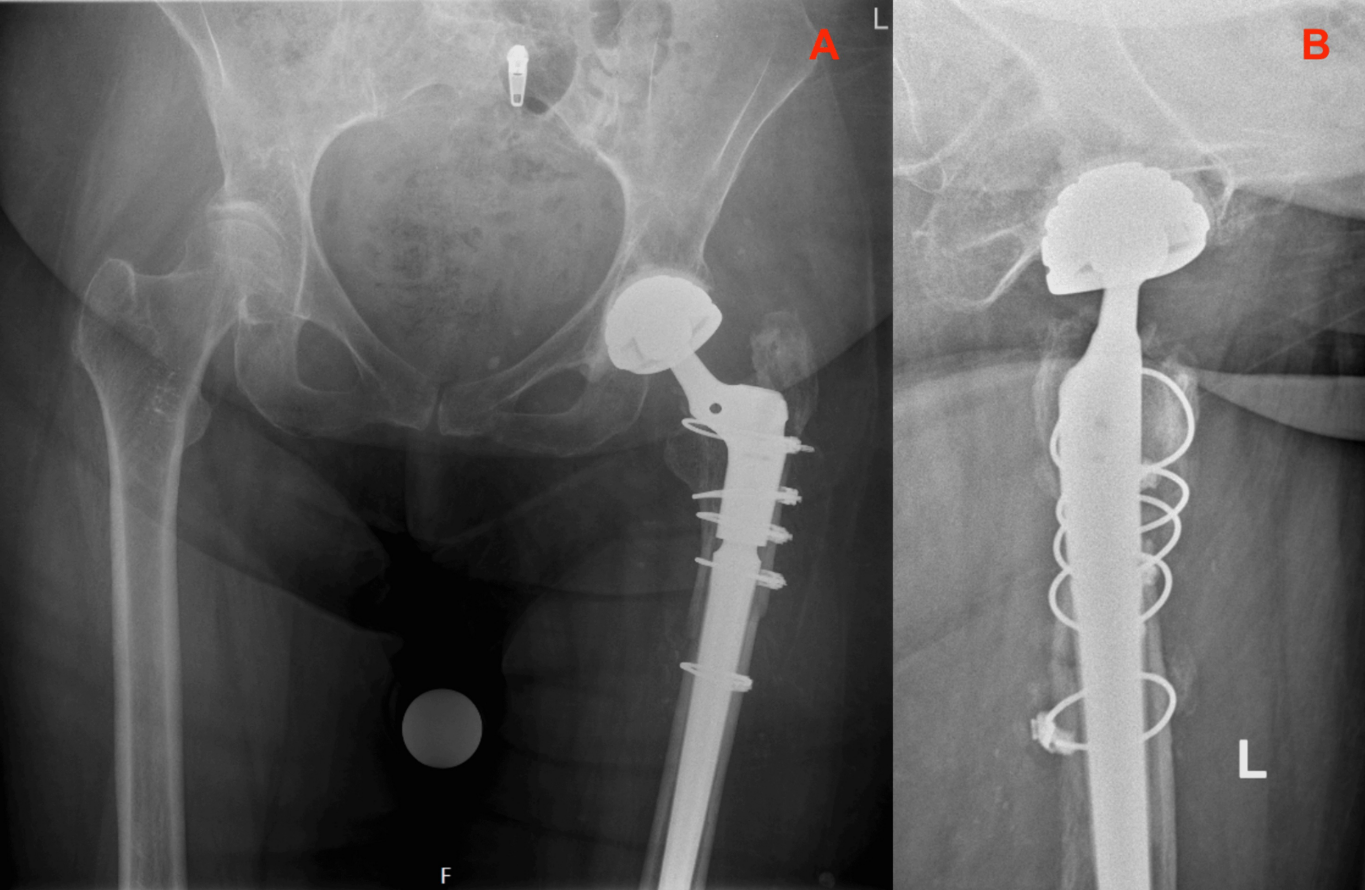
Total Hip Arthroplasty with Arcos stem and cerclages, six months after revision Six months postoperative radiographs of the revision Total Hip Arthroplasty with Arcos femoral stem and cerclages (Zimmer Biomet, Warsaw, USA), performed for a Vancouver B2 periprosthetic hip fracture. 7A: Anterior Posterior view of the Pelvis; 7B: Lateral view of the Left Hip
